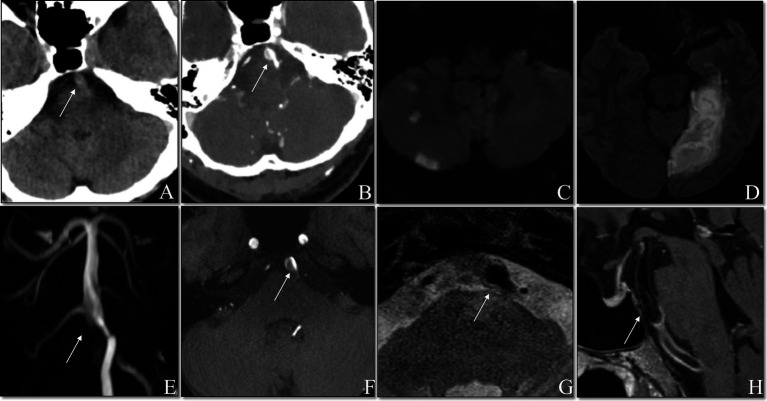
Non-contrasted cranial CT showed suspicious double lumen and intimal flap (A). Contrasted cranial CT showed the mural hematoma (B). DWI showed fresh posterior circulation infracts (C,D). TOF MRA demonstrated the basilar artery with a dissecting aneurysm (E). TOF sources showed the mural hematoma (F). Axial T2WI showed the dilated lumen of basilar artery (G). Contrast-enhanced T1WI showed an enhancement along the surface of the mural hematoma (H).
